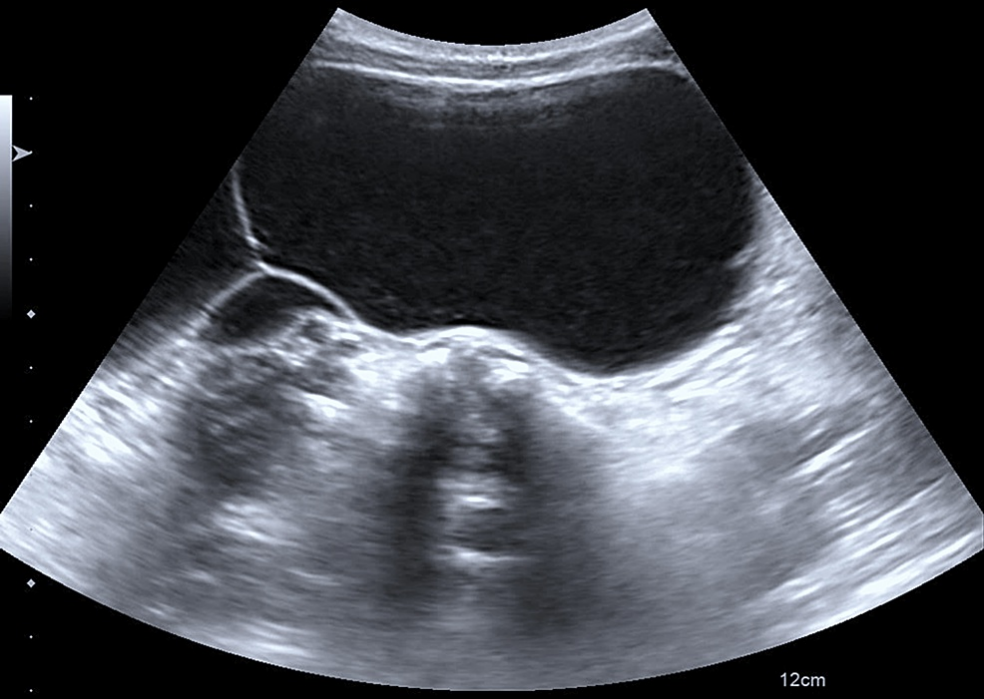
Transverse abdominal ultrasound image demonstrating multiple large multiloculated, septated cystic structures.
